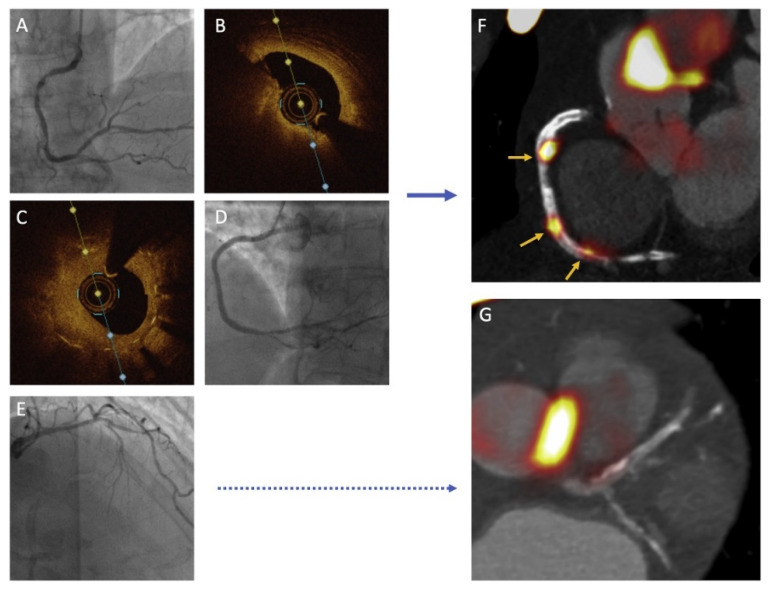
Hybrid imaging in a patient presenting with recurrent non-ST-segment elevation ACS. (A) demonstrates severe in-stent restenosis in the proximal right coronary artery (RCA) with de novo lesion in the mid-RCA; (B) OCT demonstrates plaque rupture in the mid-RCA and (C) severe neointimal hyperplasia in the previously placed stent in the proximal RCA. (D) demonstrates the result of successful revascularization, with remaining diffuse disease in the left coronary artery (E). (F,G) demonstrate the ^18^F-NaF PET-CT images with high ^18^F-NaF uptake in the culprit artery, especially in the culprit lesion in the proximal RCA. The left coronary artery demonstrates no uptake of the radioactive tracer. Reprinted from Bing et al. [141], with permission from Elsevier.
